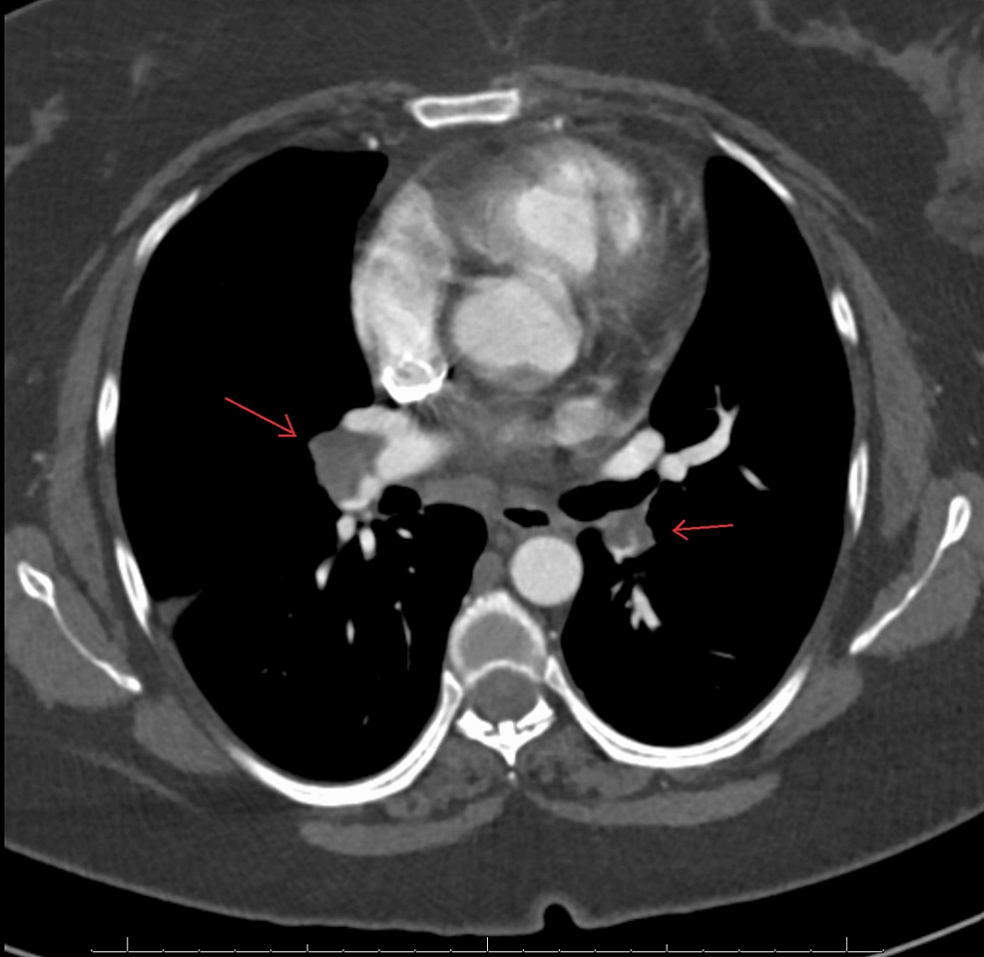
A CTA of the chest in axial view demonstrates acute bilateral pulmonary emboli involving the right pulmonary artery distally with extension into some of the right lower lobe and right middle lobe segmental and subsegmental arteries and some of the left lower lobe and left upper lobe segmental/subsegmental arteries and mildly enlarged pulmonary arteries. CTA: computed tomography angiography
